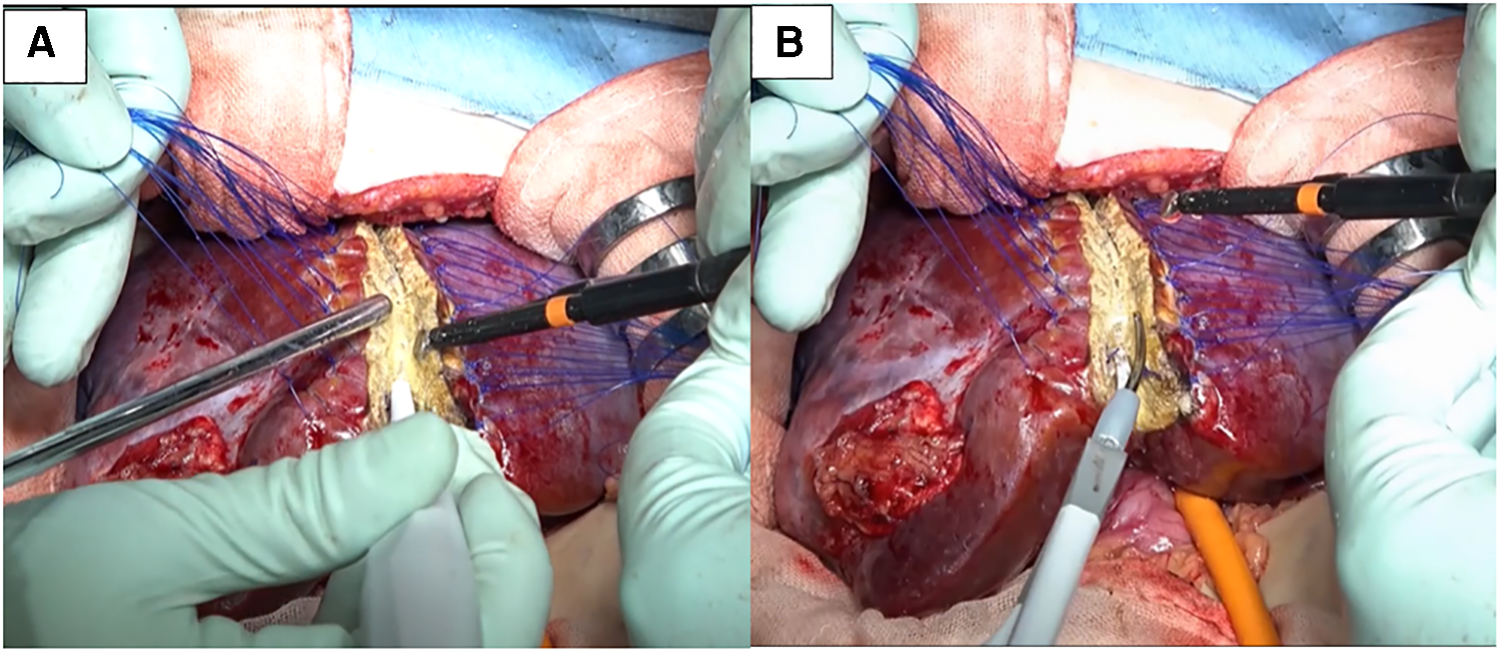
(A) We used a water-jet dissector for dissection of the parenchyma. (B) Vessels >5 mm were resected with an ultrasonically activated device after division using a water-jet dissector.
